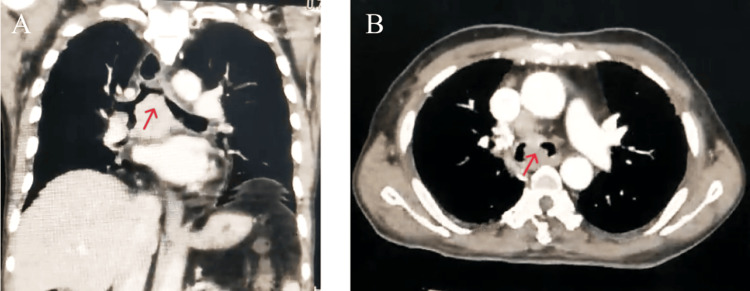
Computed tomography of the chest demonstrating a central airway tumor (arrow) at the level of the carina in the coronal view (A) and axial view (B)
